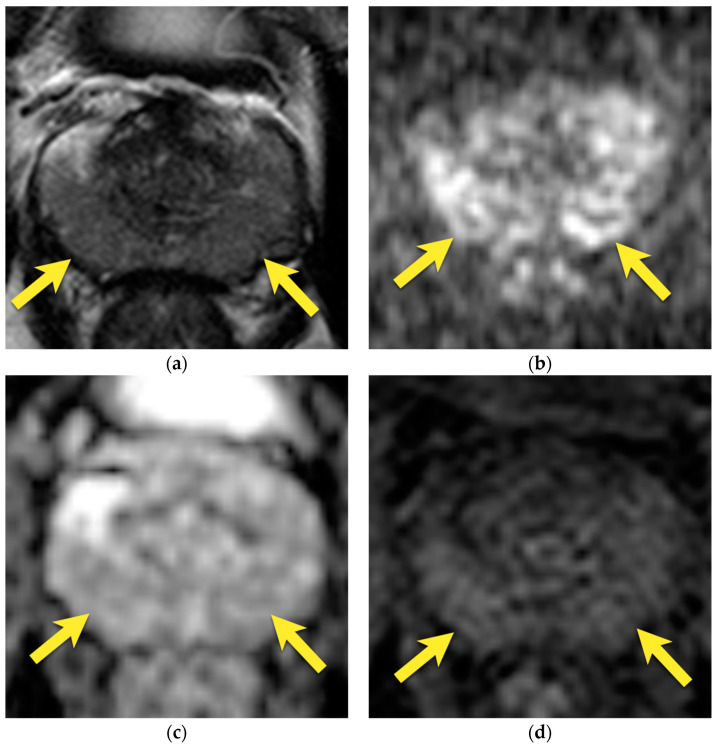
Prostatitis in a 48-year-old patient with elevated PSA 5 ng/mL: (a) axial T2-w image shows diffuse, decreased signal in the peripheral zone (arrows), with moderate diffusion restriction; (b) diffuse, moderately increased signal on DWI b 2000 image (arrows); (c) diffuse, moderately decreased signal on ADC map (arrows); (d) mild, diffuse enhancement of PZ (arrows). Findings reported as PI-RADS 2.
